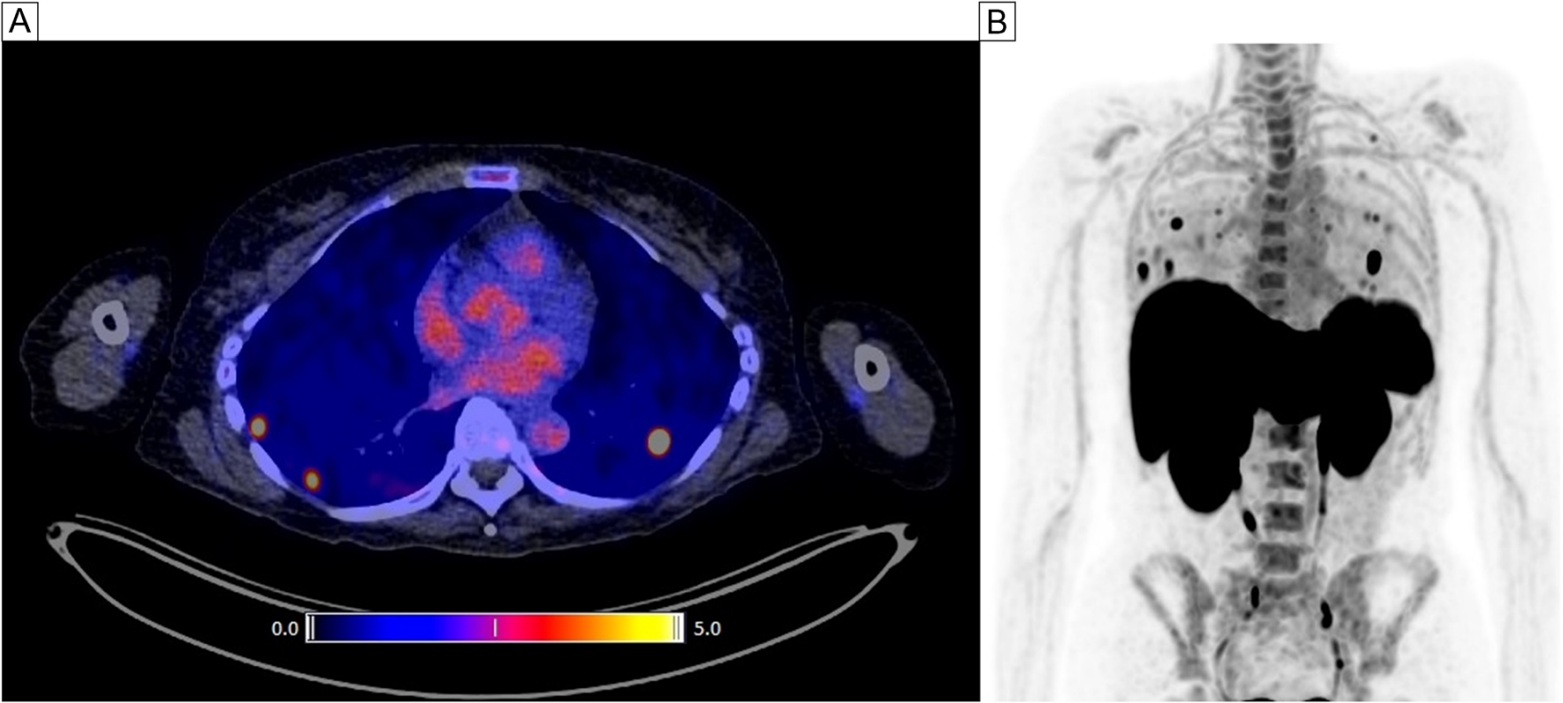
Example of high tracer uptake in the lungs. A Transversal PET/CT image of three regions showing high [^18^F]FB-IL2 uptake. B Maximum intensity projection of the same patient showing multiple areas of high tracer accumulation in the lungs
